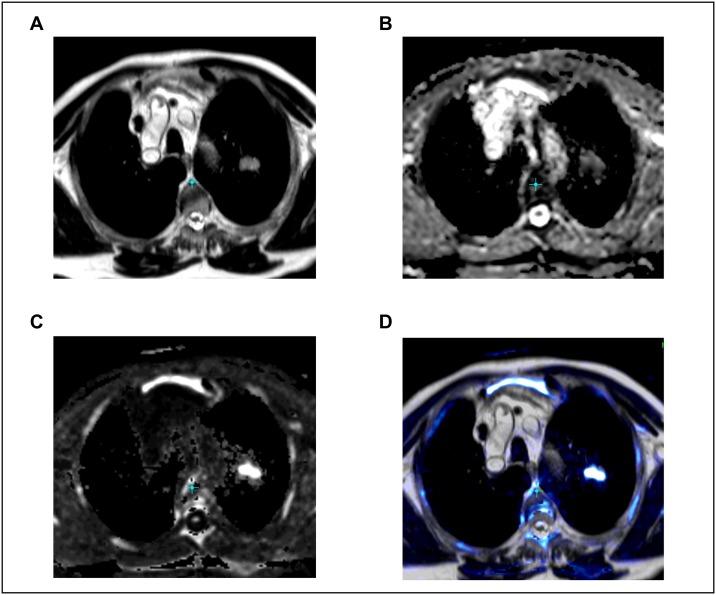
MRI sequences of a patient with stage T1b NSCLC in the left upper lobe. A. T2 HASTE sequence (non-gated). B. ADC-map. C. DWI (calculated b-value of 1400). D. Fusion of T2 and DWI, color represents diffusion restriction.
